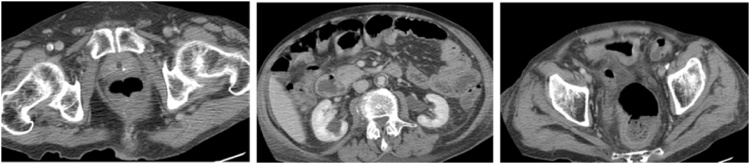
A repeated computed tomography ten days after the first CT. Left panel: Shows resolution of fecaloma; Middle panel: Shows resolution of the small bowel distension; and Right panel: Shows persistent wall thickening and air within bladder.
